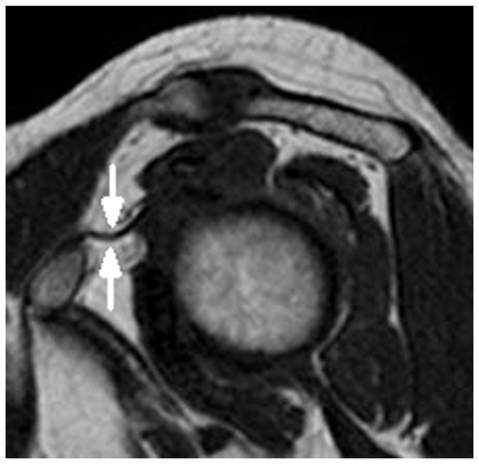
A 49-year-old male normal volunteer. Sagittal oblique, T1-weighted image (TR/TE = 550 ms/15 ms) demonstrates a normal CHL showing homogeneous , low signal intensity (arrows).
